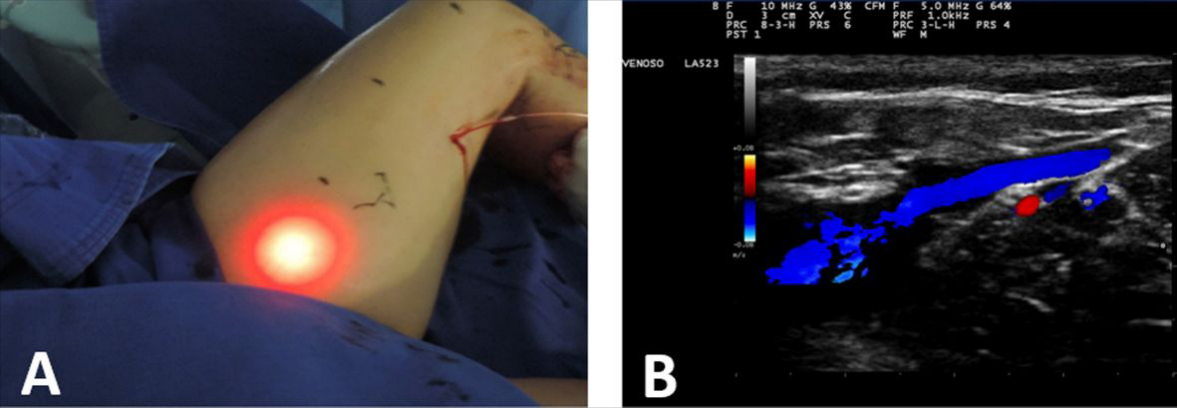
Confirmation of fiber position by transillumination (A) and ultrasound control (B).
